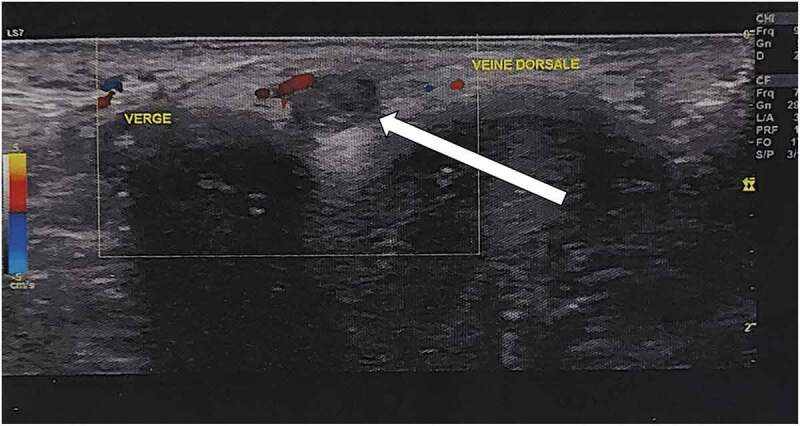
Doppler ultrasound evalution showing no flow in the vein.
